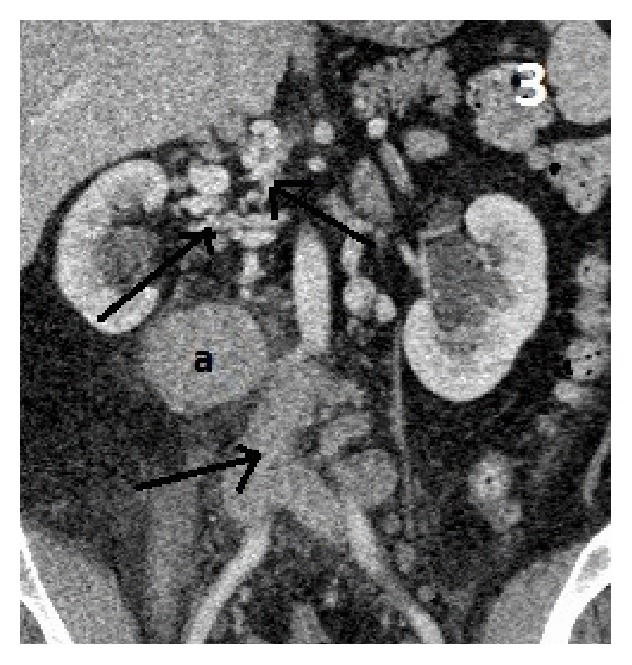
Abdomen CT coronal view: (a) IVC venous varix. Arrows pointing enlarged, multiple retroperitoneal/paralumbar collateral veins.
